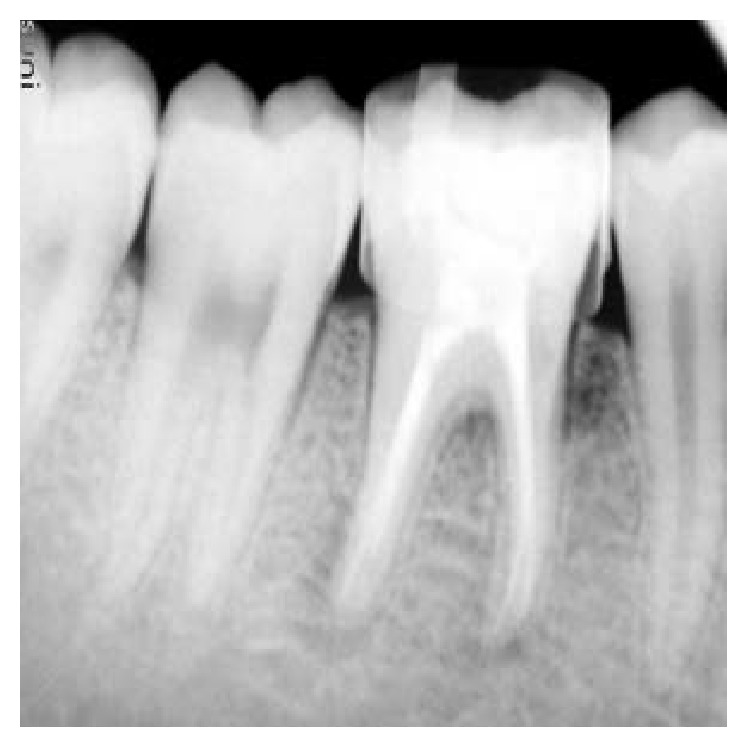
Postobturation radiograph of 46.
